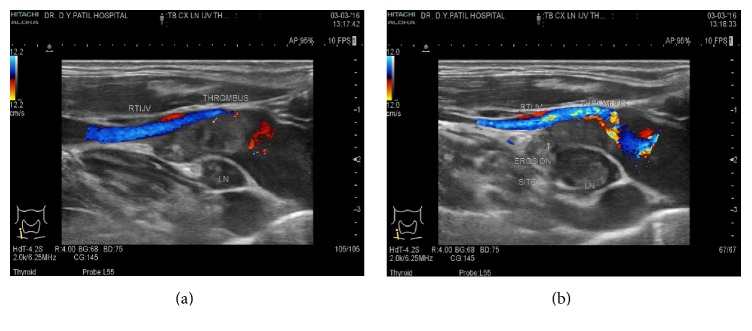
Color Doppler study showing IJV thrombus seen as filling defect.
